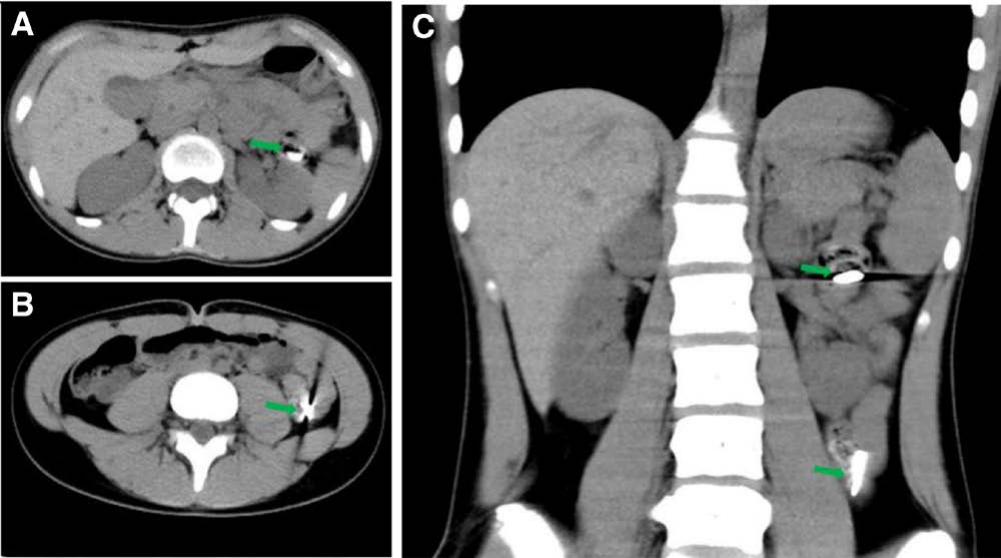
Abdominal CT showed 2 dense shadows in the transverse and descending colon (A–C). CT = computerized tomography.
